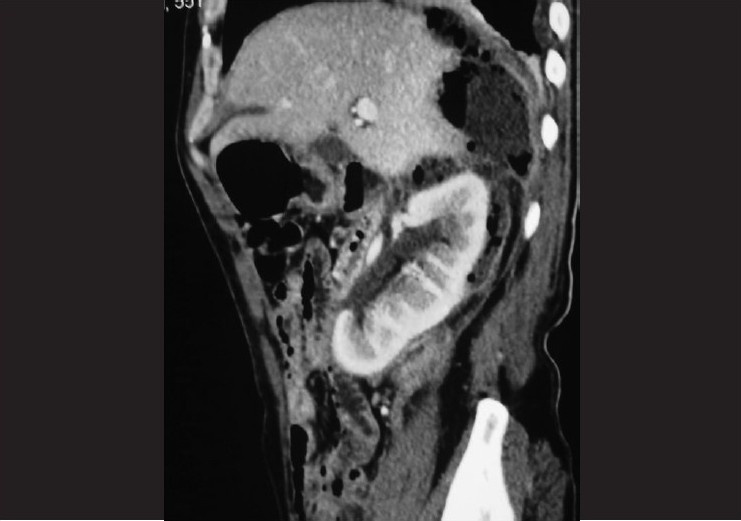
Sagittal reformat CT image showing right perinephric abscess reaching superiorly upto bare area of liver with inflammed appendix at its lower end below right kidney
